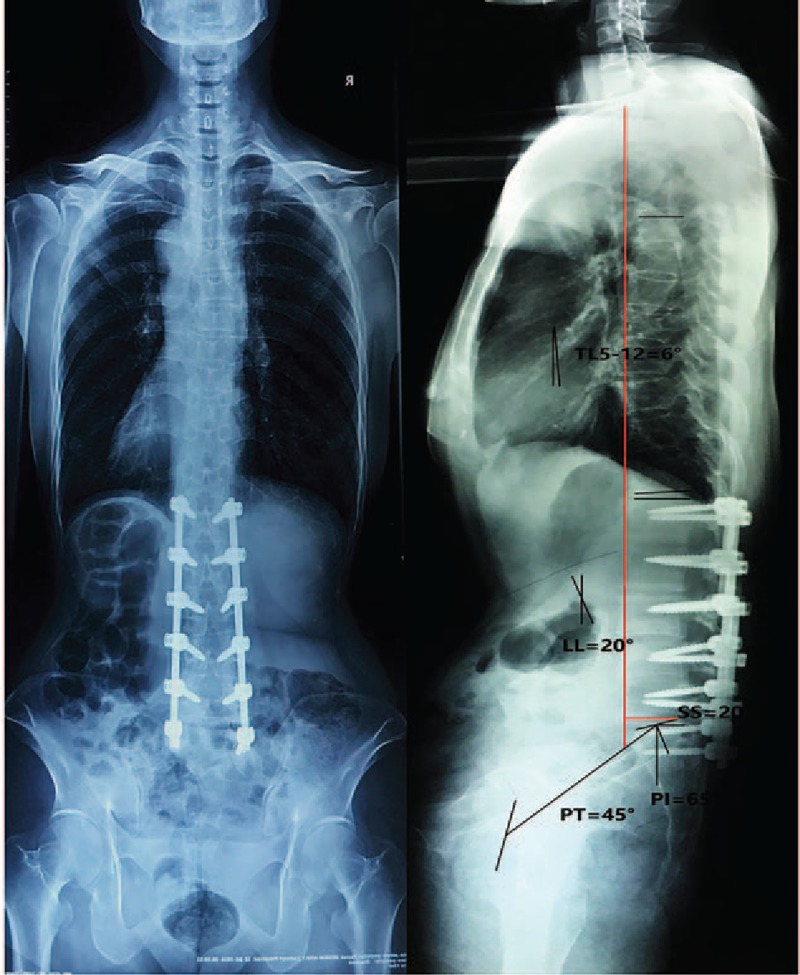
Positive and lateral full spine x-ray 1 year after surgery. PI = 65°, PT = 45°, SS = 20°, LL = 20°, T5-T12 = −6°, SVA = 36 mm. PI = pelvic incidence, PT = pelvic tilt, SS = sacral slope, SVA = sagittal vertical axis from C7 plumb line.
